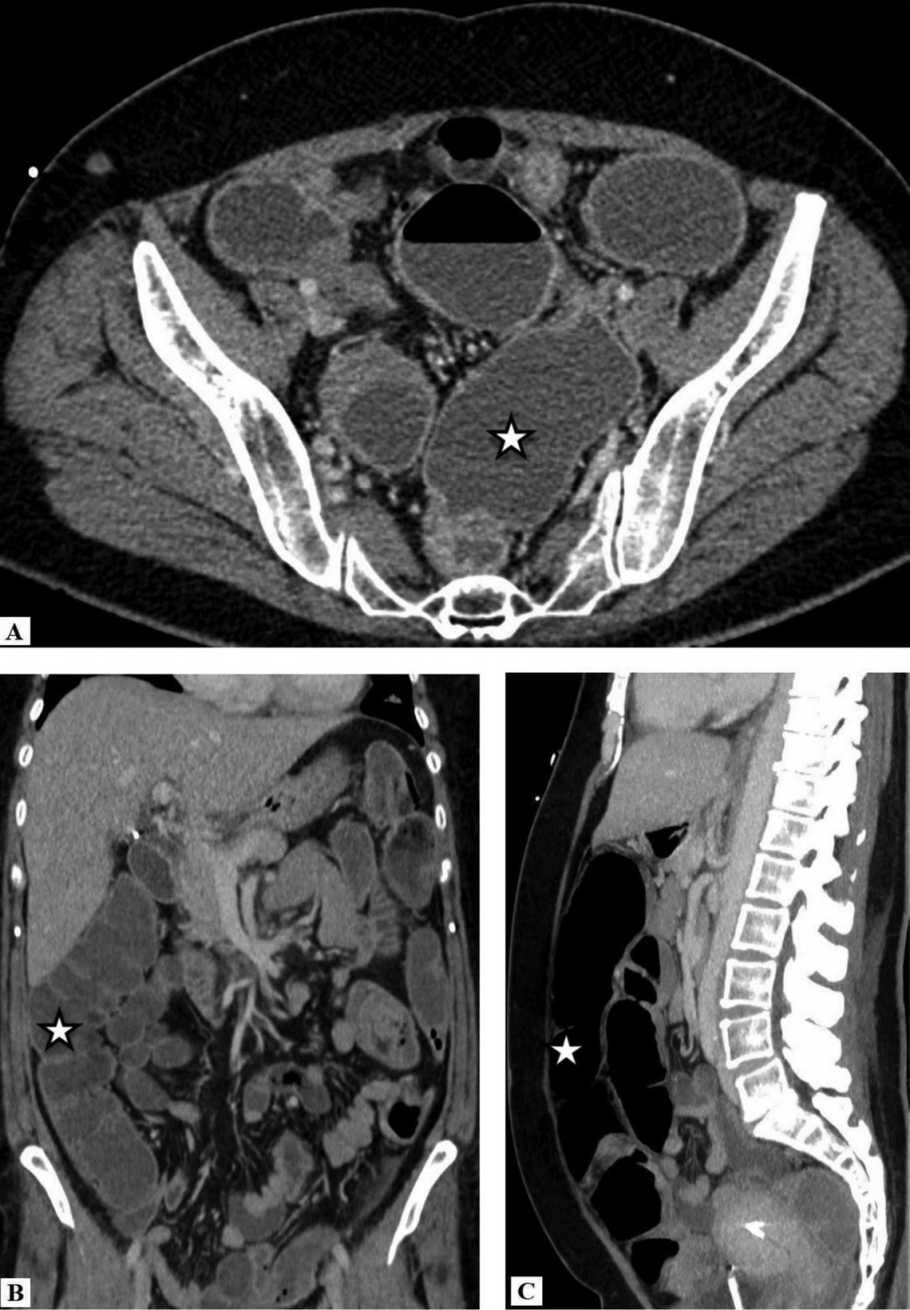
Contrast-enhanced computed tomography (CECT) scan in the (
